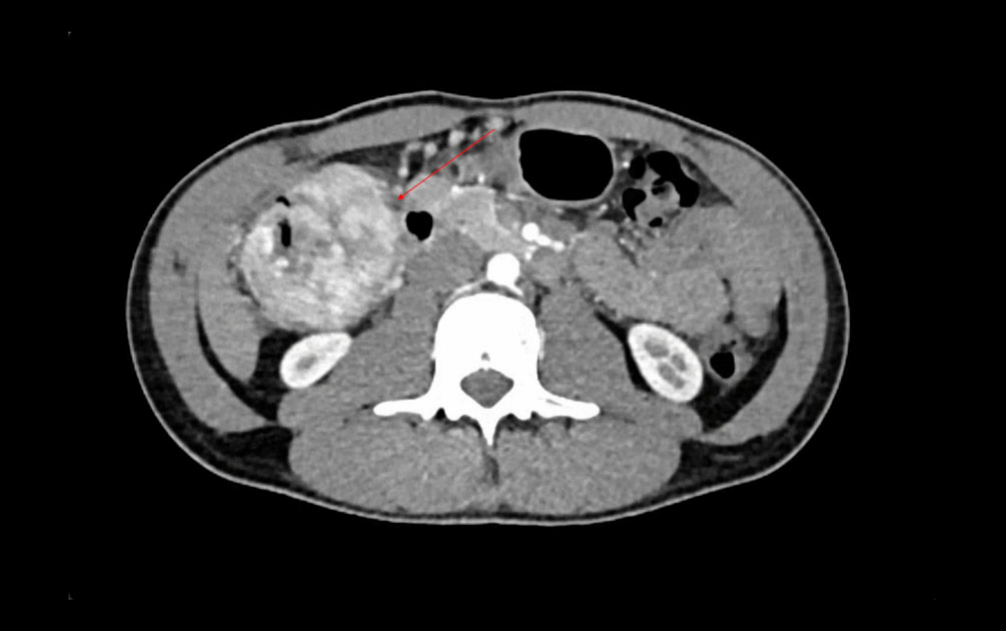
Computed tomography scan performed 1 month later demonstrating mobility of the lesion.
